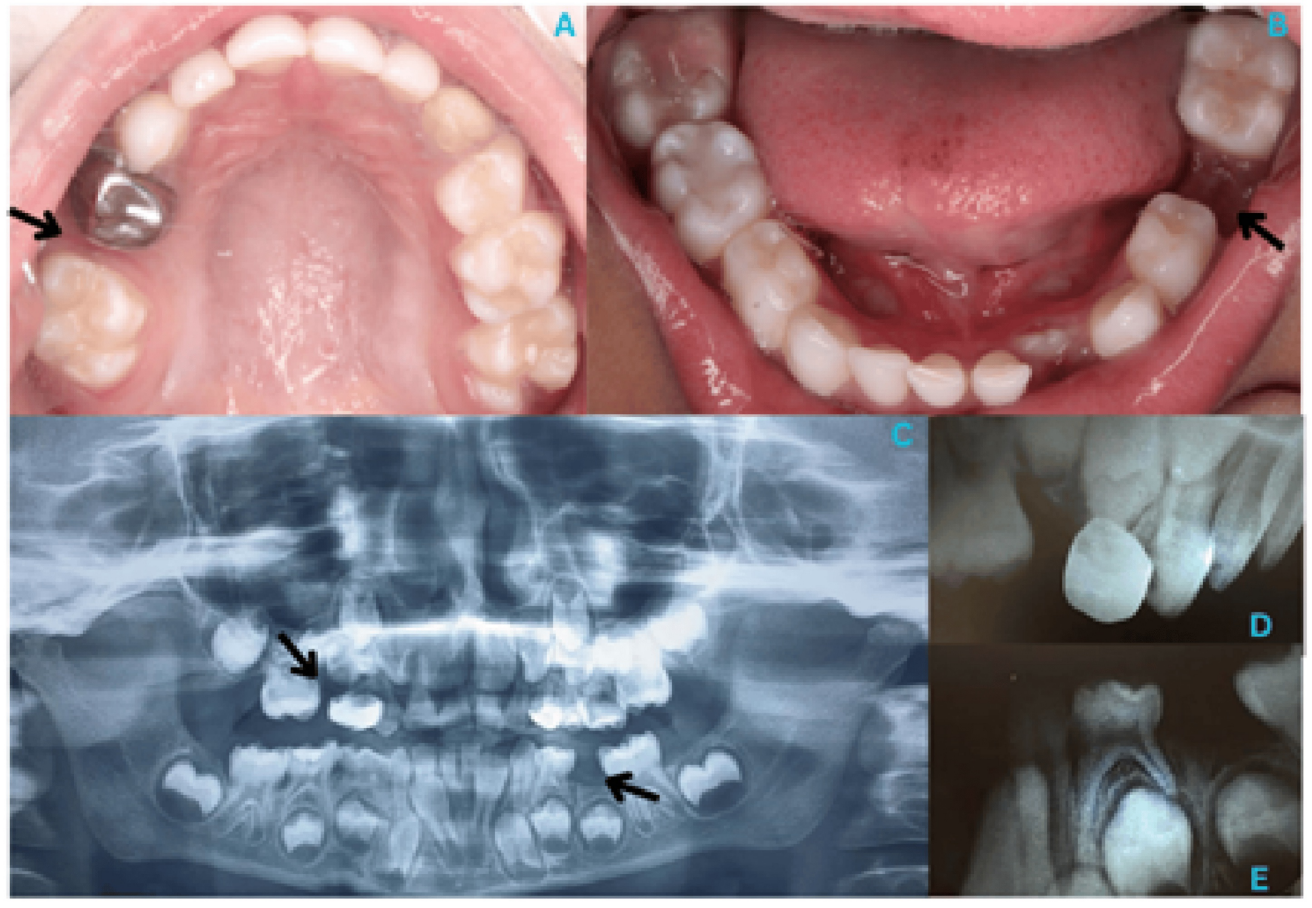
Preoperative Photograph and Radiographs (A) Preoperative maxillary occlusal view. (B) Preoperative mandibular occlusal view. (C) Orthopantomogram - Huckaba analysis revealed a space loss of 3 mm in the region of tooth 55 and 0.7 mm in the region of tooth 75. (D) Intraoral periapical radiograph in relation to tooth 55. (E) Intraoral periapical radiograph in relation to tooth 75.
